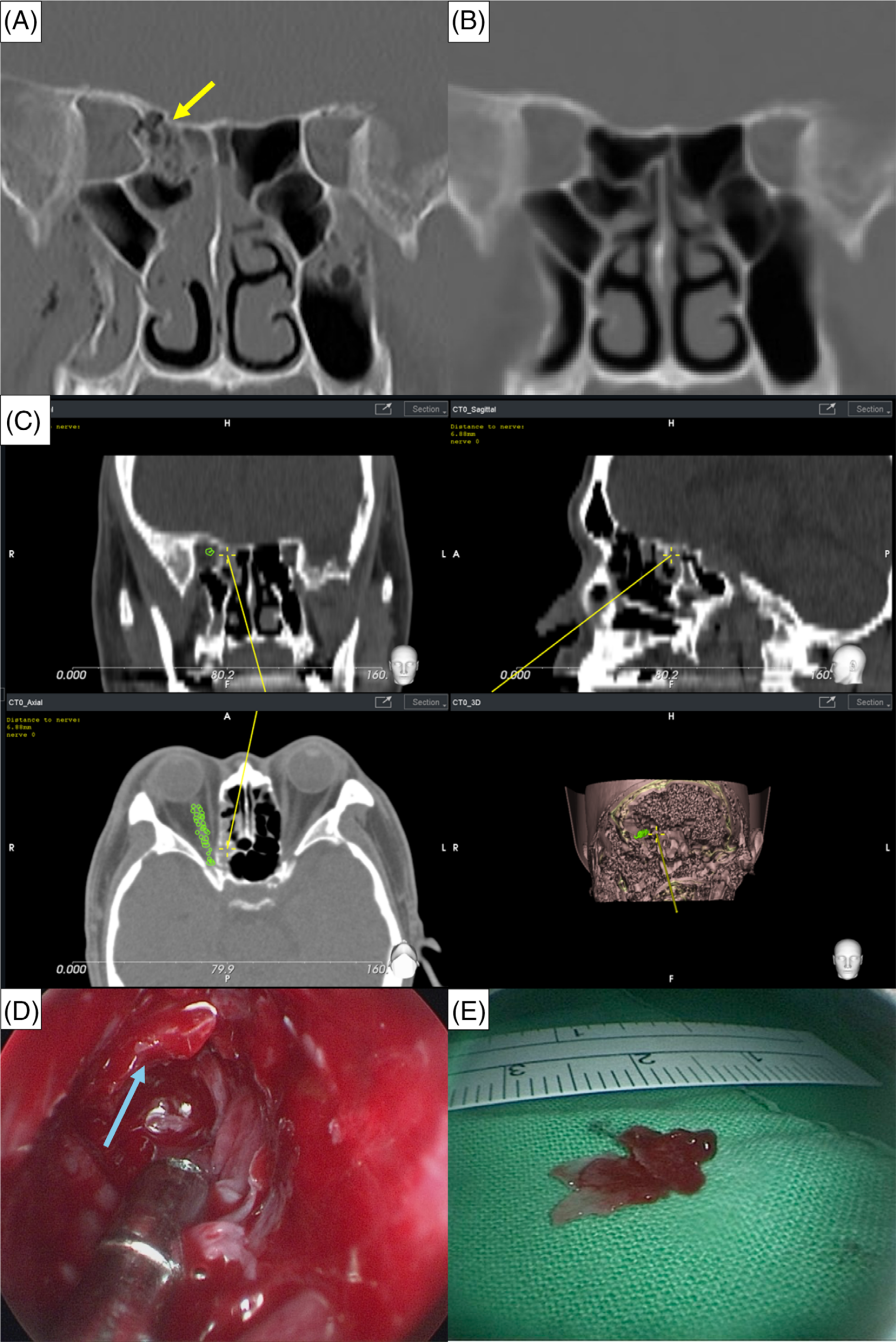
(A) Preoperative coronal CT scan. The yellow arrow indicates bony fragments impinging upon the right optic nerve. (B) Postoperative coronal CT scan. (The left eye served as a reference for lesion location, Figure S1). The scan confirmed successful removal of bony fractures and decompression of the optic nerve. (C) Navigation of instrument tip (yellow cross), marked at a distance of 6.88 mm from the optic nerve (Figure S2). The navigation system is programmed to provide warnings if the tip approaches within 5 mm of the optic nerve, minimizing the risk of iatrogenic injury. (D) Endoscopic view captured from the right nostril depicting ethmoid bone fragments (light blue arrow). (E) This bone fragment seen in 1D was removed, measuring up to 1.3 × 0.7 cm in size.
